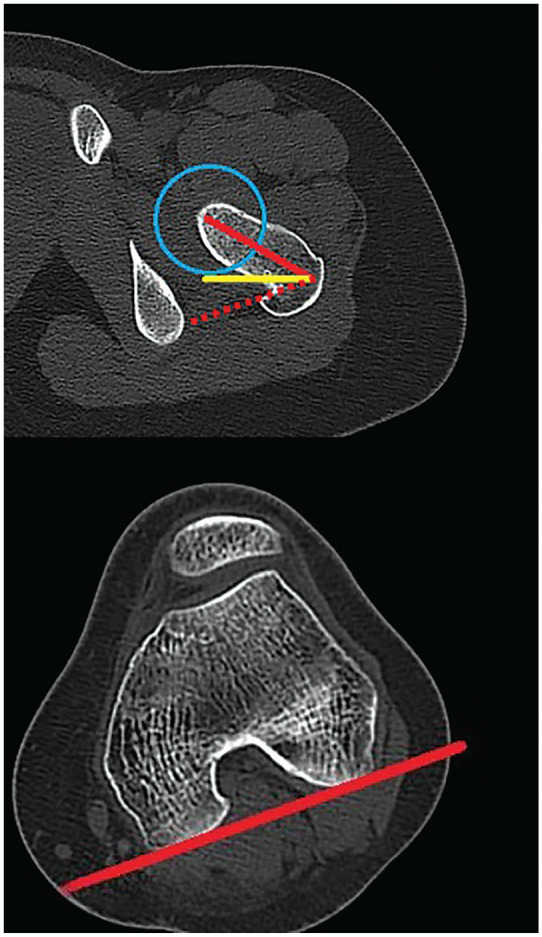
Measurement of femoral torsion in preoperative computed tomography scans of a left (upper) proximal femur and (lower) distal femur in the axial plane. The center of the femoral head was defined. The red line in the upper scan shows the femoral neck axis. The yellow line in the upper scan determined the horizontal plane. The red line in the lower scan and the dotted line in the upper scan represent the parallel line to the posterior femoral condyles. The angle between the dotted line and the red line in the upper scan represents the femoral torsion.
